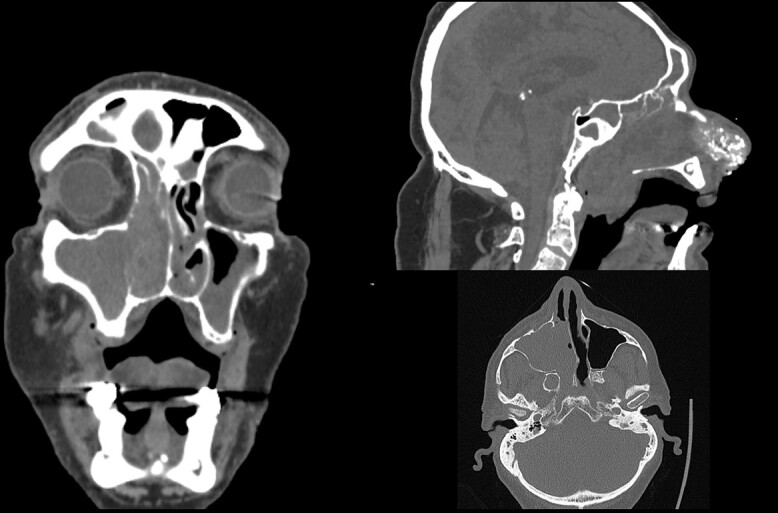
Initial CT scan of the head and neck region in coronal, axial and sagittal view show expansive formation predominantly in the right half of the paranasal sinuses. Right maxillary sinus is completely filled with tumor mass as well as right nasal cavity with infiltration of the septum, all ethmoid cells and the frontal sinus through frontoethmoid recess. Sagittal view show tumor spread in the nasopharynx.
